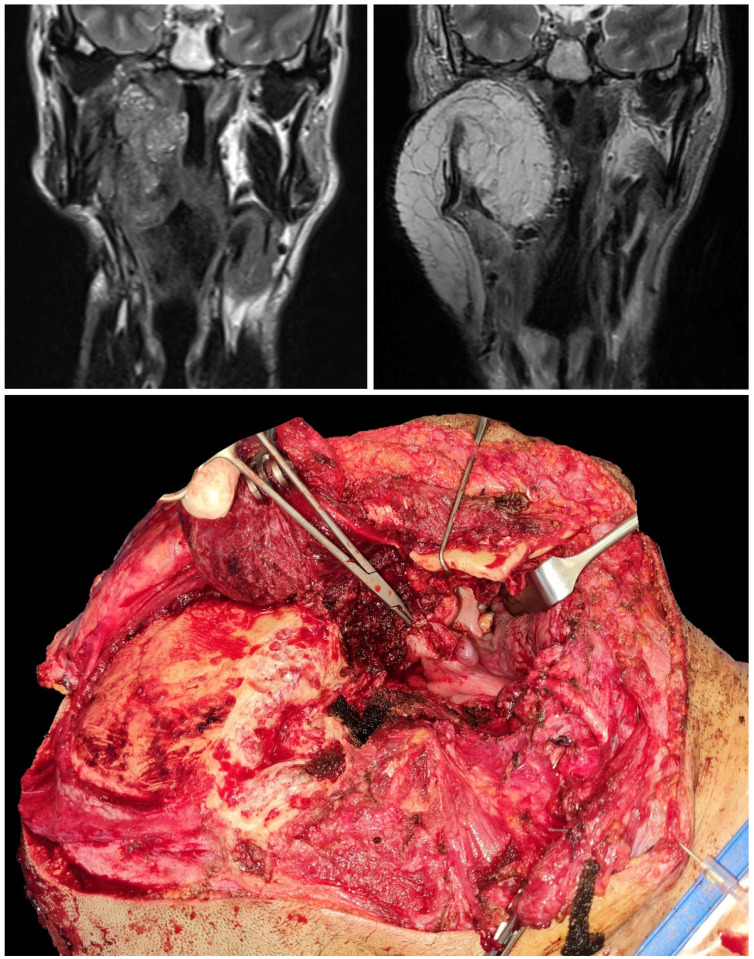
Pre-operative (upper left image) and post-operative (upper right image) magnetic resonance imaging of a patient affected by relapsing NUT carcinoma of the right parotid. Intraoperative (lower image) appearance of the surgical field after the ablation phase, which included a temporo-parotid resection [16] extended to the parapharyngeal space, masticator space, lateral nasopharyngeal and oropharyngeal walls, and middle cranial base.
